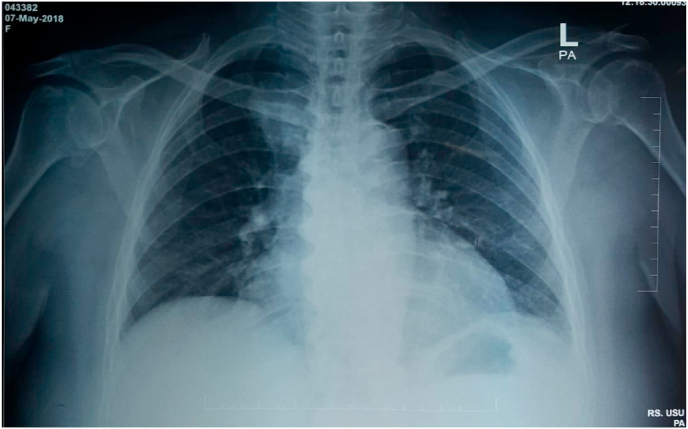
Plain Chest X-Ray of 68 years old woman with mediastinal angiolipoma. Plain Chest X-Ray showed a right paratracheal mass (arrows) with a well-demarcated lesion attached to the mediastinal cage.
